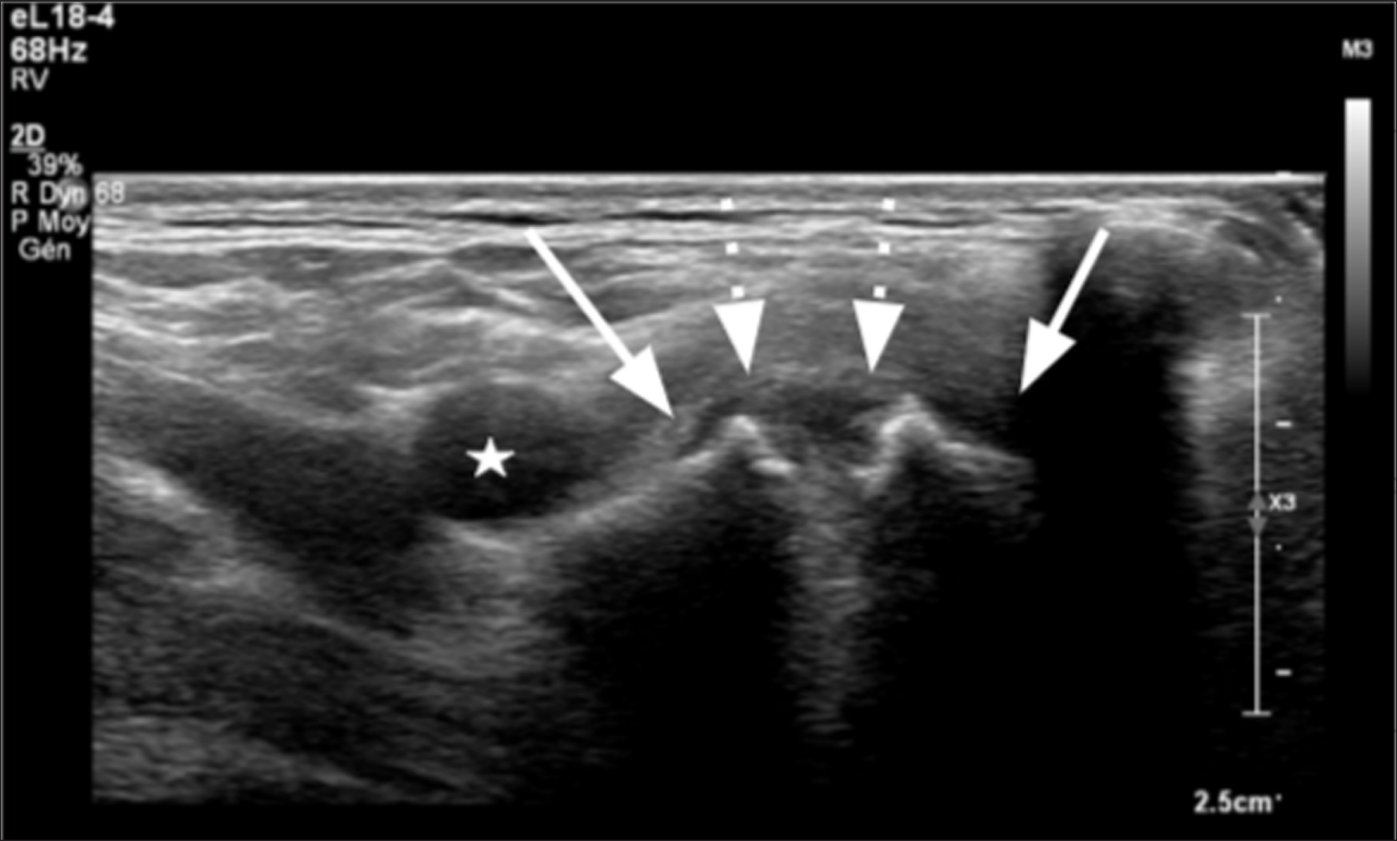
Cervical and comparative X-rays of the clavicular region were performed, showing a right complete cervical rib (*Figure 2*, plain arrow), forming a pseudarthrosis (*Figure 2*, arrowhead) with a bony protrusion from the lateral arch of the first right rib (*Figure 2*, dotted arrow) A shorter left cervical rib was also present (*Figure 2*, white star).
